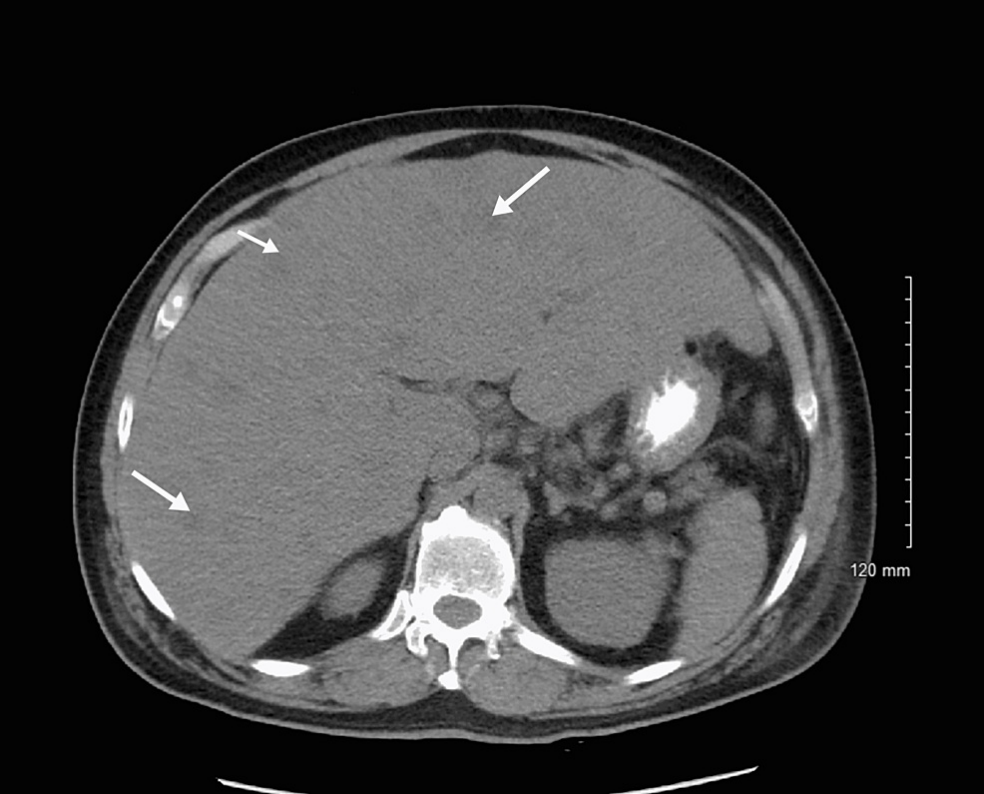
CT abdomen and pelvis showing hepatomegaly with numerous metastasis (white arrows).
